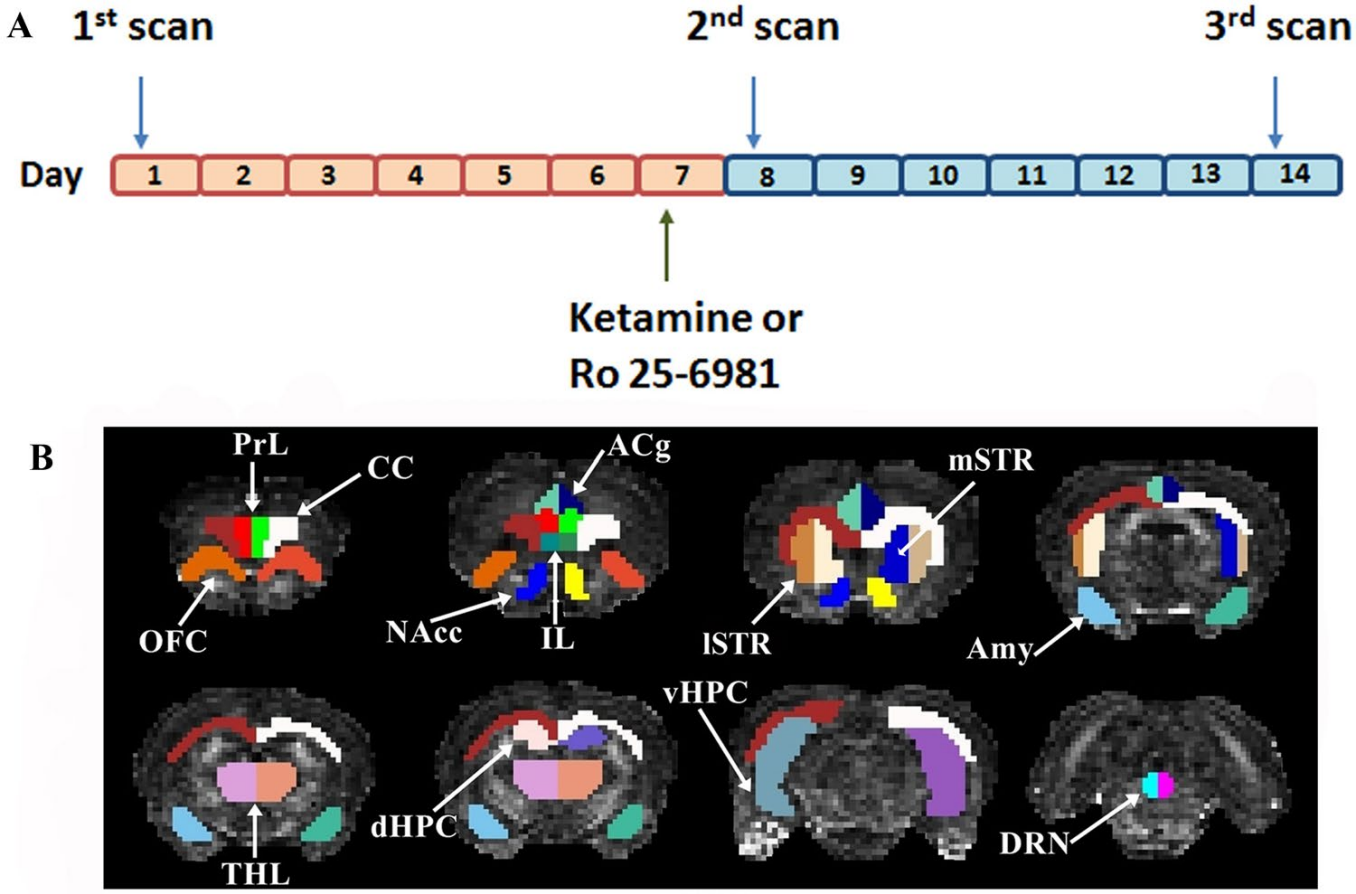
Experimental procedure for DWI analysis. A Timeline for DWI scans. B Coronal DWI images showing pseudo-colored, hand-drawn regions of interest (ROIs). Abbreviations: *PrL* prelimbic cortex; *CC* corpus callosum; *OFC* orbitofrontal cortex; *ACg* anterior cingulate cortex; *IL* infralimbic cortex; *NAcc* nucleus accumbens; *lSTR* lateral striatum; *mSTR* medial striatum; *Amy* amygdala; *THL* thalamus; *dHPC* dorsal hippocampus; *vHPC* ventral hippocampus and *DRN* dorsal raphe nucleus
